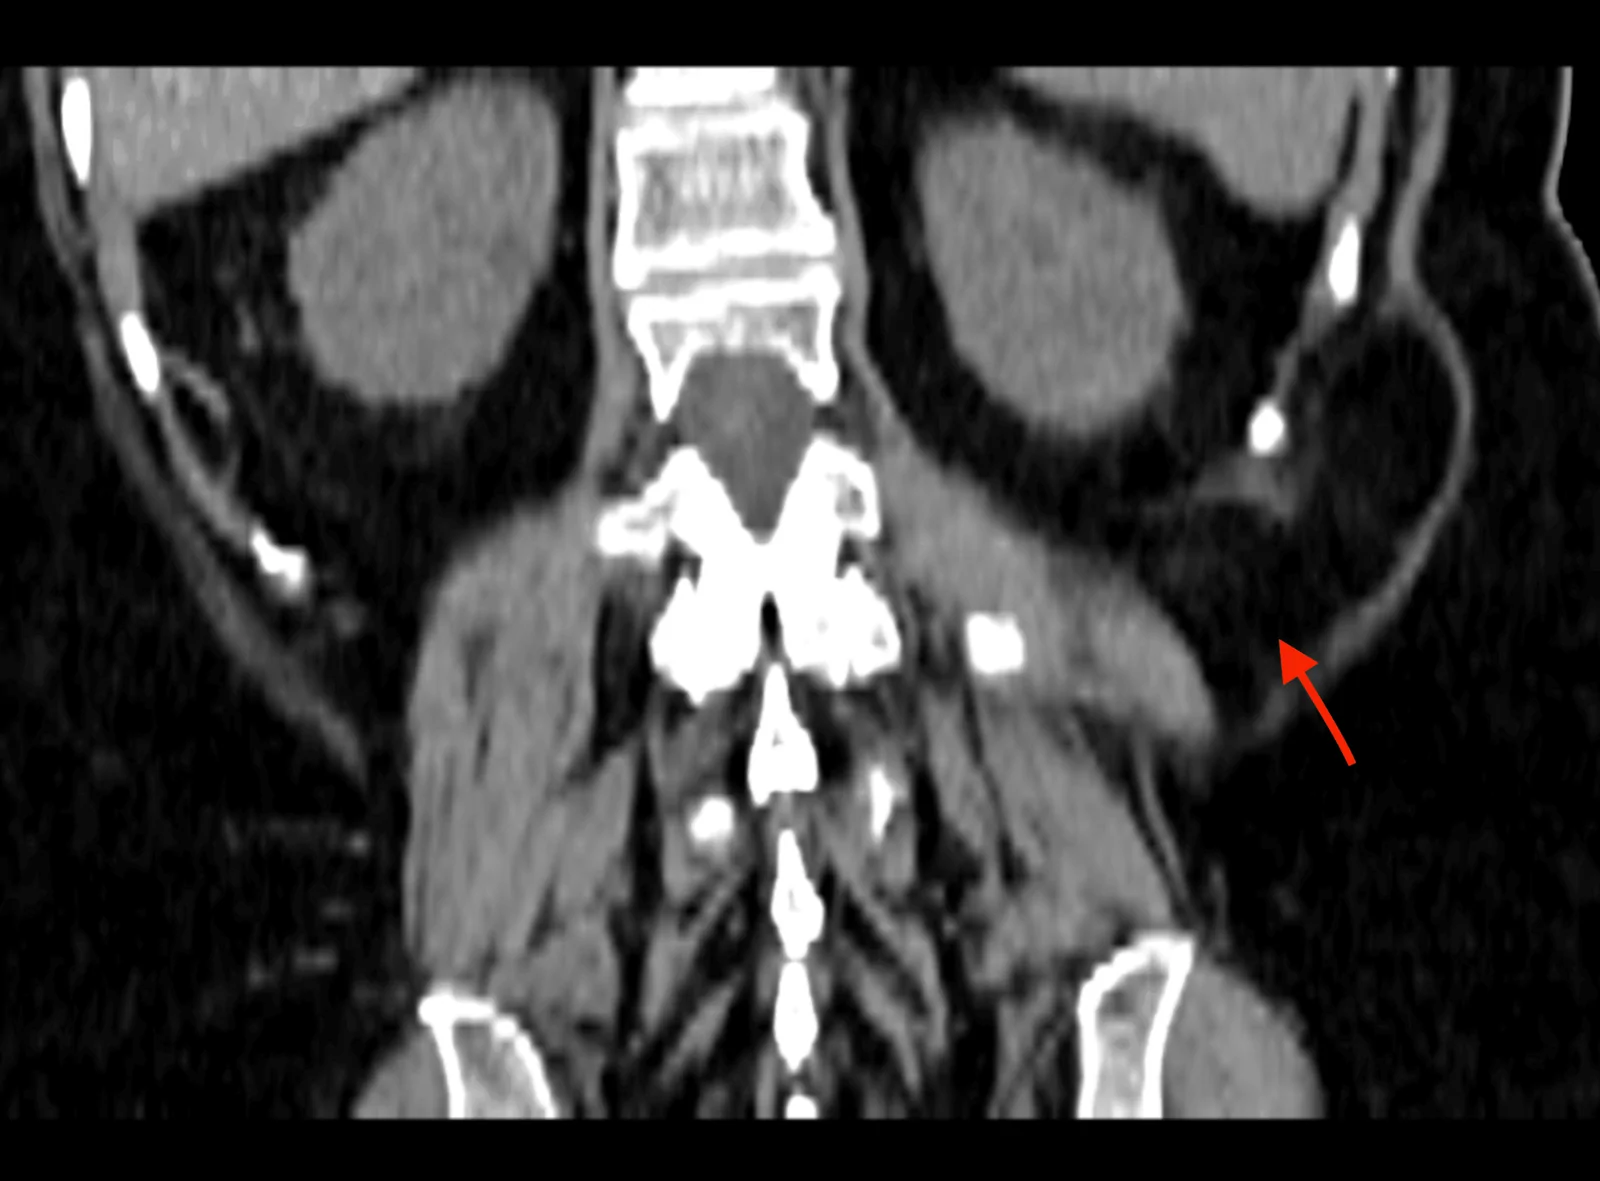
Non-contrast abdominal CT, coronal reformation Superior lumbar triangle defect (arrow) with retroperitoneal fat herniation, without visceral involvement or signs of incarceration.
